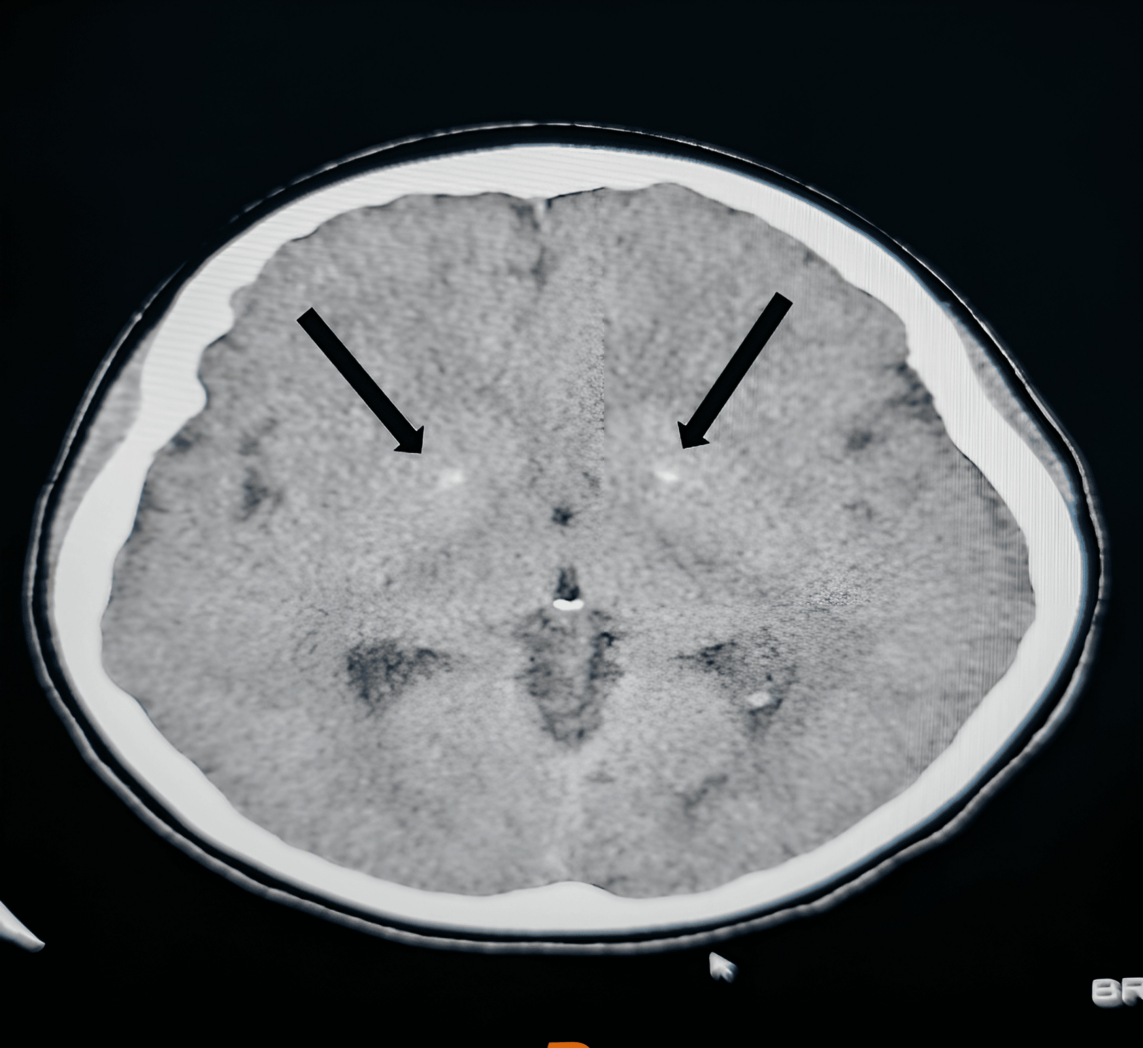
CT brain shows basal ganglia calcification (arrows).
